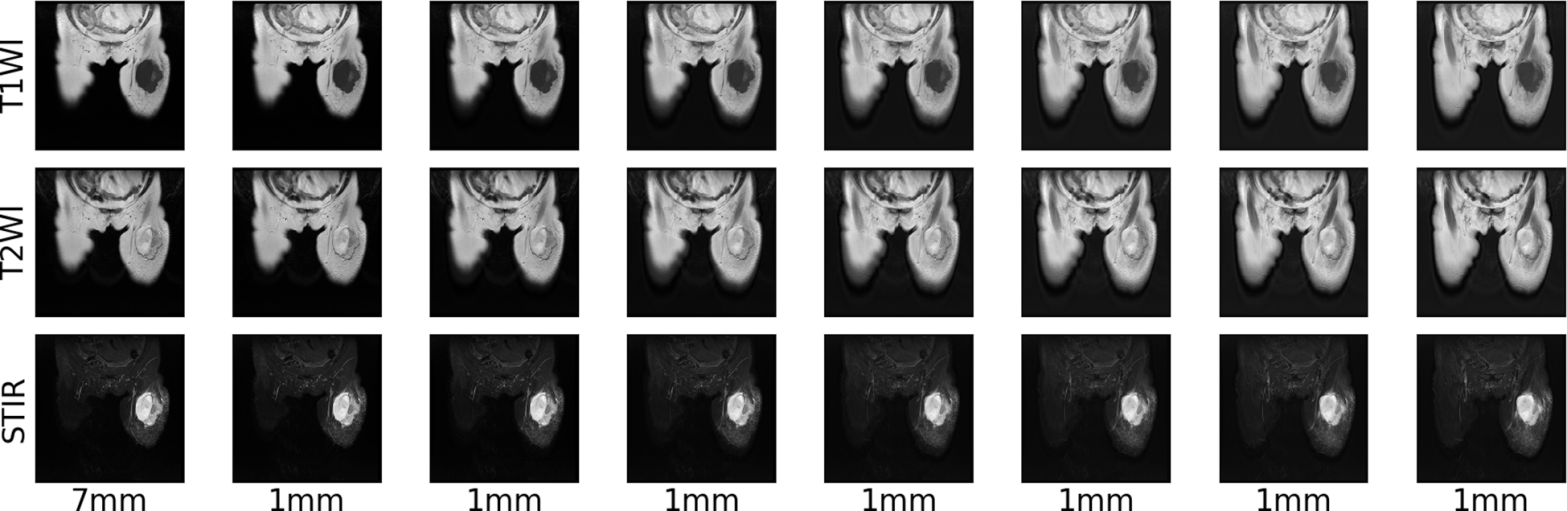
MRI resampling: 9 mm thickness of each modality of the original MRI image of a patient with a soft tissue sarcoma, 1 mm thickness of each modality of the MRI image after interpolation resampling.
